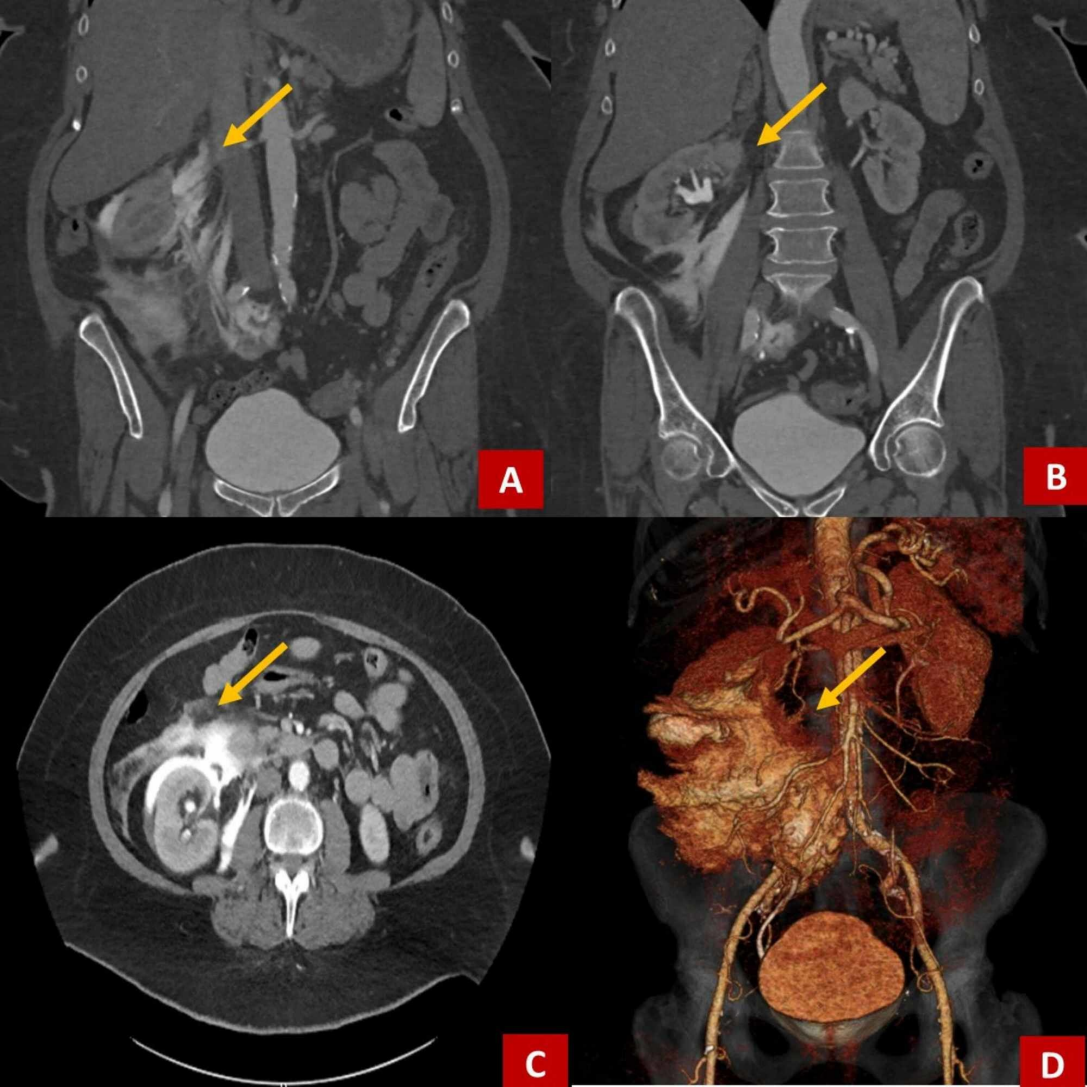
CT abdomen and pelvis with angiography coronal and transverse planes. Demonstrates fluid in the upper right quadrant near the kidney, stomach, and duodenum. This imaging suggested renal or urethral origin of the fluid suspected to be a urinoma at the time of disposition. A,B: coronal plane, C: transverse plane, D: 3D reconstruction.
